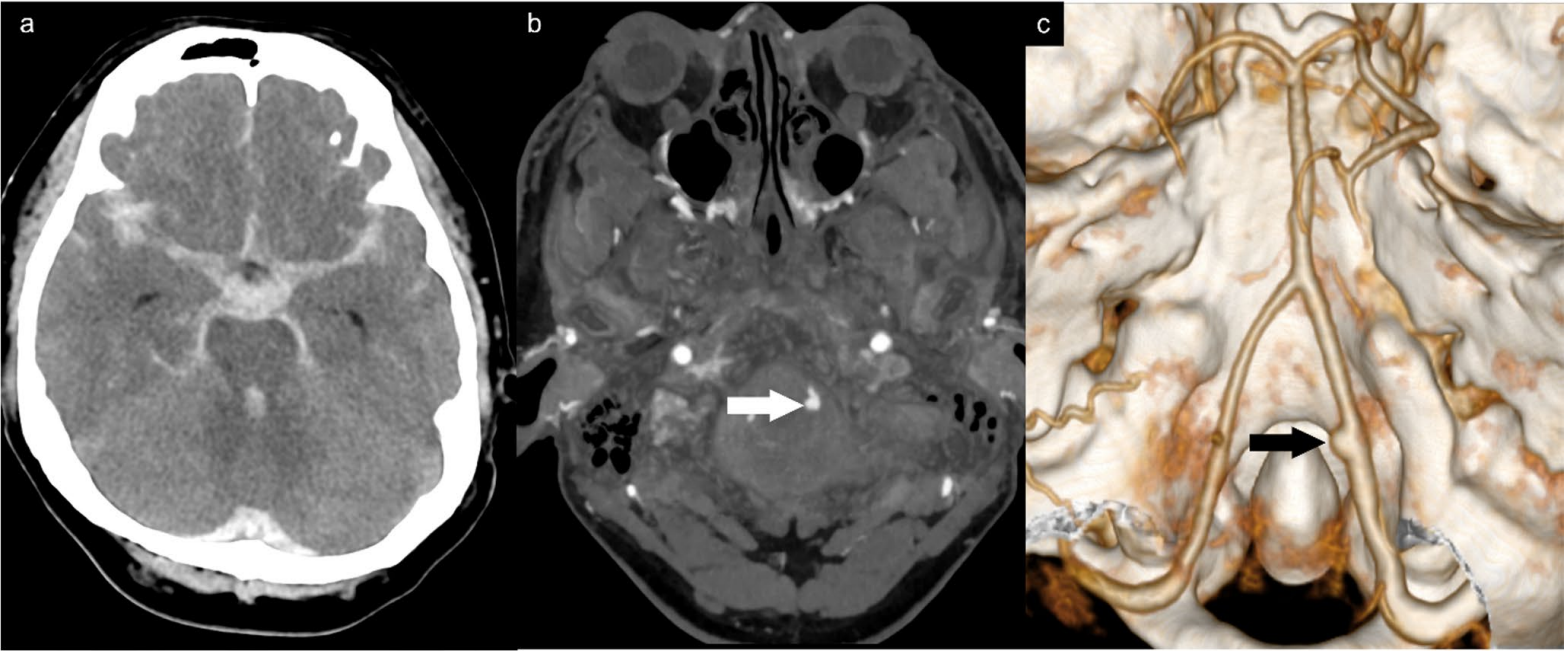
Left VA pseudoaneurysm (a) NCCT shows massive SAH and IVH. (b) 0.2-mm CTA demonstrates an outpouching vascular lesion at the medial wall of the mid-V4 segment of the left VA (arrow), which is detected on the VRT image (c) (arrow)
